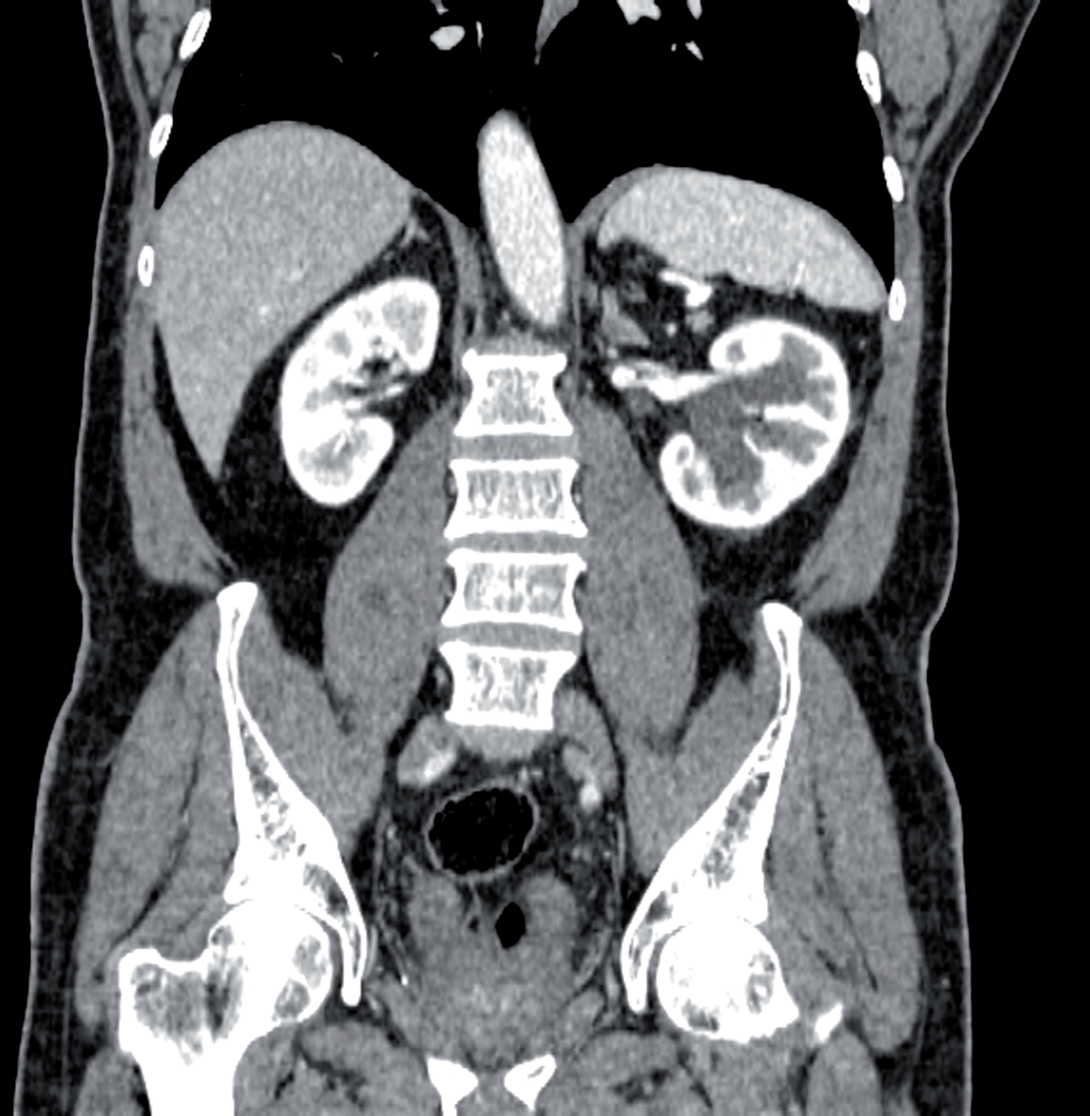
Coronal view of computed tomography at the nephrographic phase two years after the operation. One observes mild to moderate left pyeloectasis and good cortical contrast uptake.
